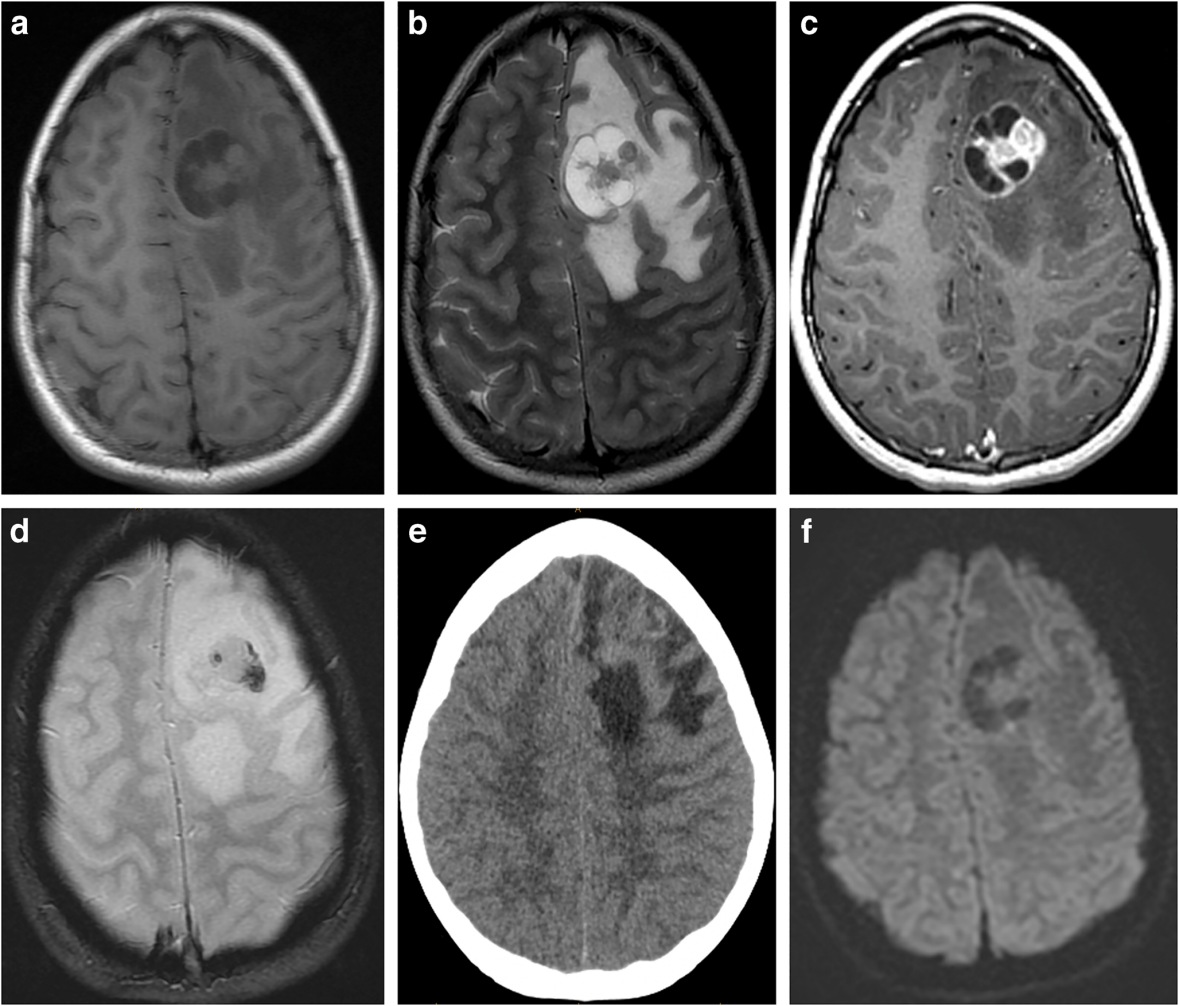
Pleiomorphic xanthoastrocytoma. a An axial non-contrast T1-weighted scan through the frontal lobes demonstrates a solid cystic tumor in the left frontal lobe. b Axial T2-weighted image through the same level better shows the solid cystic tumor. Extensive peritumoral T2 abnormality is also visible. c Axial postcontrast T1-weighted image through the same level better demonstrates enhancement of the solid component and the septae of the tumor. d Axial GRE image through the same level better demonstrates susceptibility artifact from intratumoral hemorrhage as there is no calcification within the tumor on CT (e). There is also no diffusion restriction on diffusion-weighted sequence (f)
